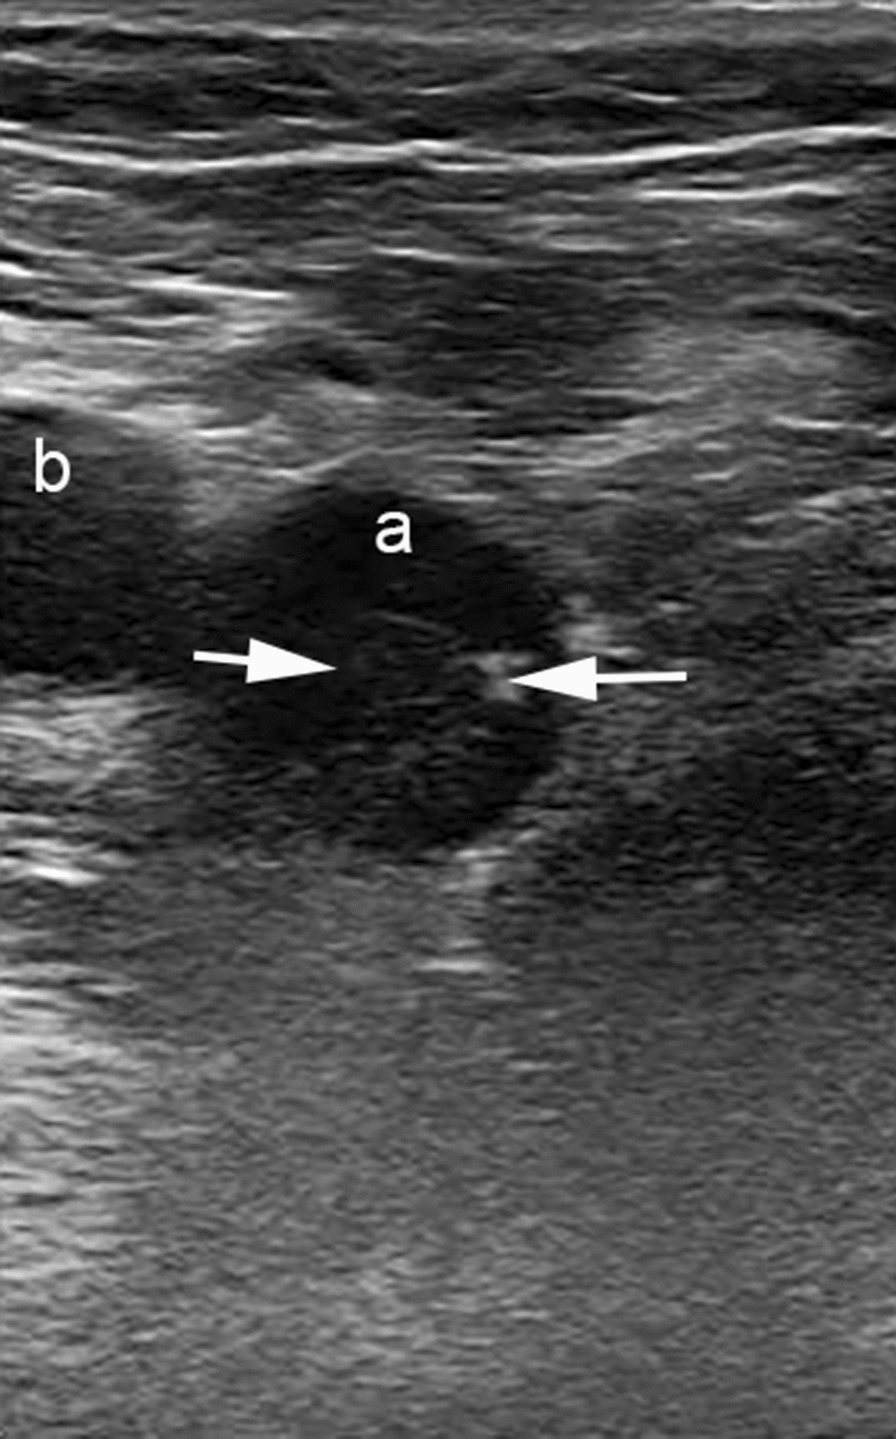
Ultrasound image of a person with compression ultrasound confirmed DVT. Short axis ultrasonographic view of the right femoral vein (a) showing thrombus (arrows) attached to the wall. The right superficial femoral artery (b) is seen adjacent to the femoral vein. DVT: deep vein thrombosis
